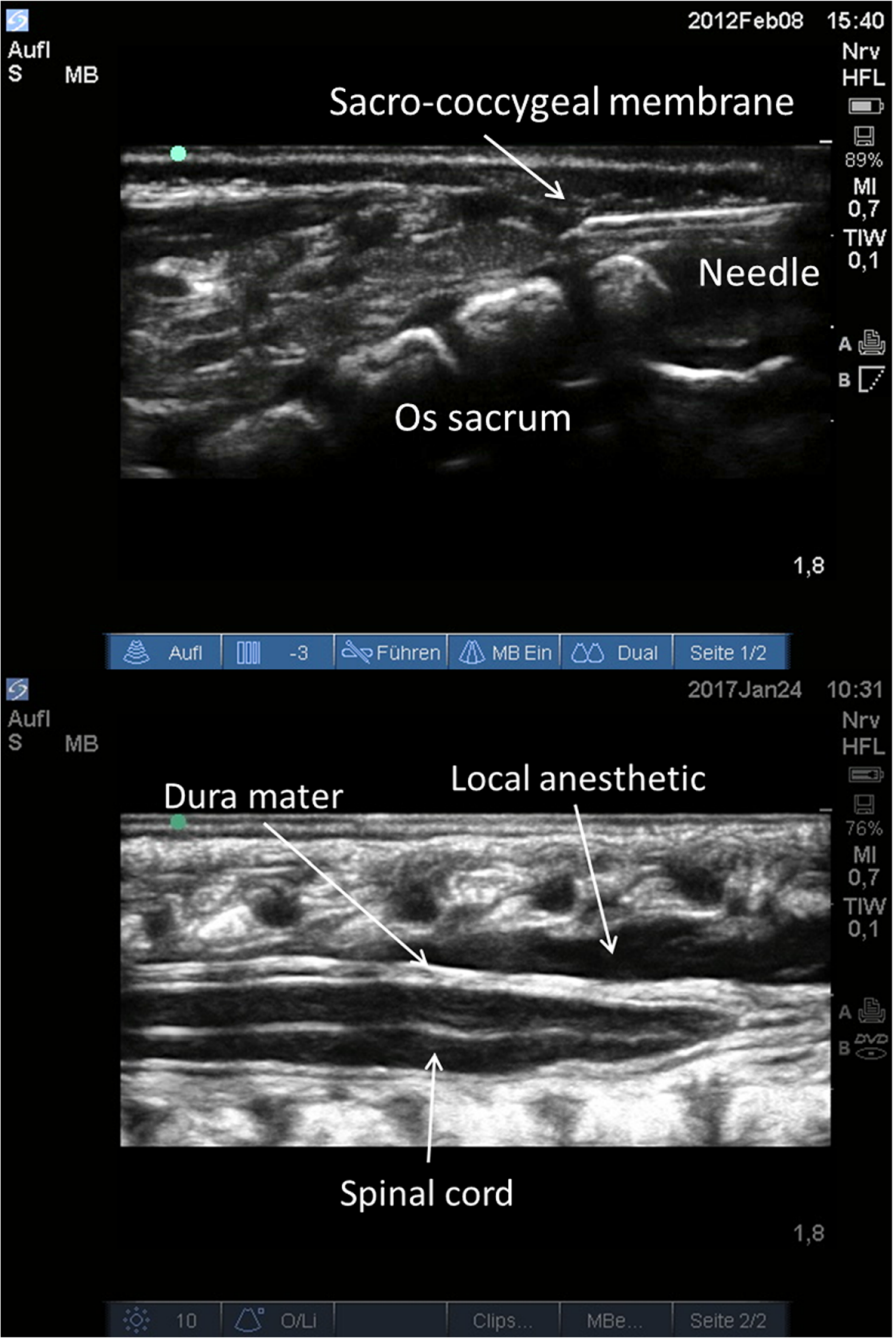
Ultrasound images of the implementation of caudal anaesthesia in very low birth weight infants scheduled for open inguinal hernia repair. Caudal anaesthesia was implemented in infants placed in a lateral decubitus position with the left side down. Correct needle placement and distribution of the local anaesthetic was observed in all patients as visible on the ultrasound image and led to a surgically sufficient analgesia. Both pictures show a long axis view of the spinal canal. a Puncture with an Epican®Paed25G was performed in an in-plane technique by means of a SonoSite® M-Turbo with linear probe (5–13 MHz). b The ultrasound picture in in-plane technique demonstrates the spread of the local anaesthetic (ropivacaine 0.375%; 1 ml/kg)
